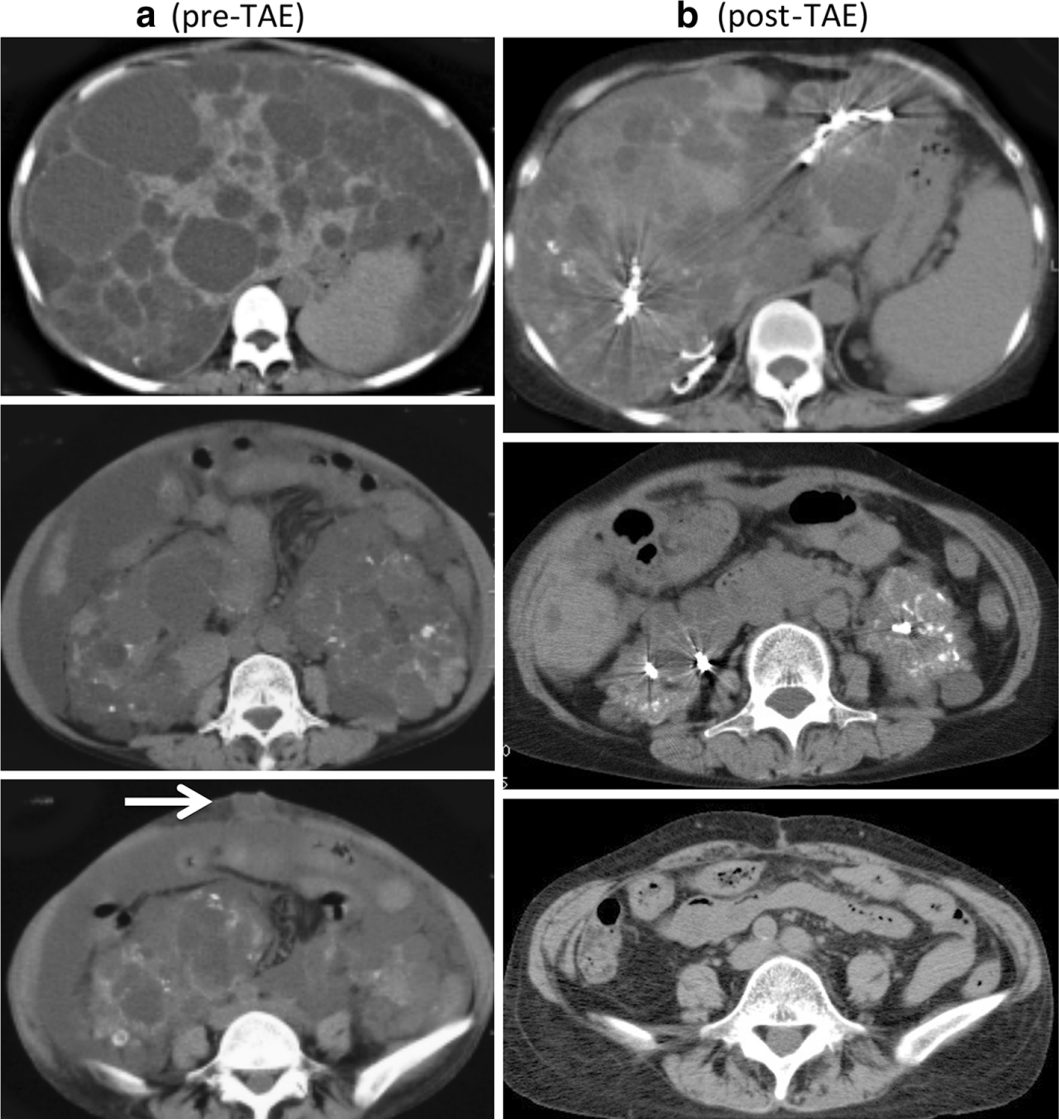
a Computed tomography images pre-TAE. b Computed tomography images post-TAE. *Arrow* shows protruded umbilical hernia
